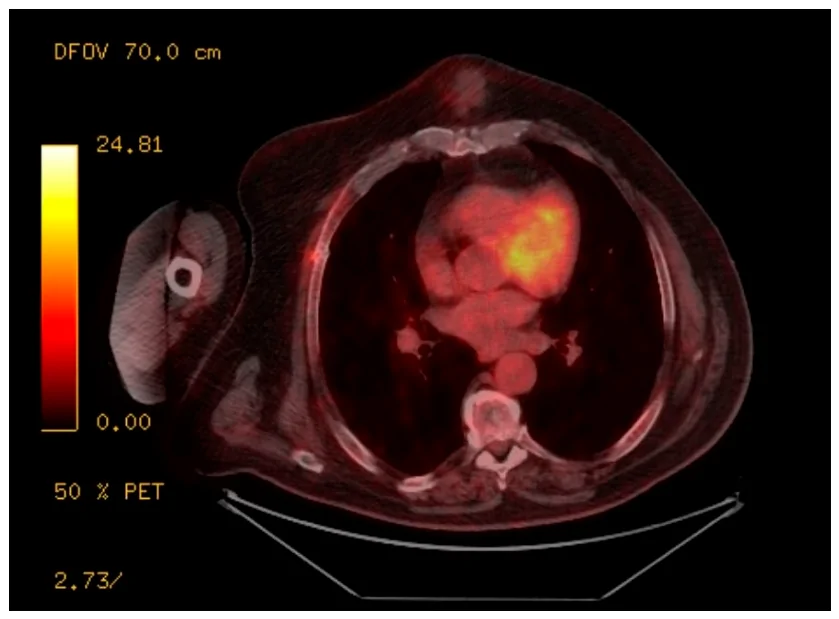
Preoperative axial fused PET/CT caption through the lower thorax at the level of the heart.
